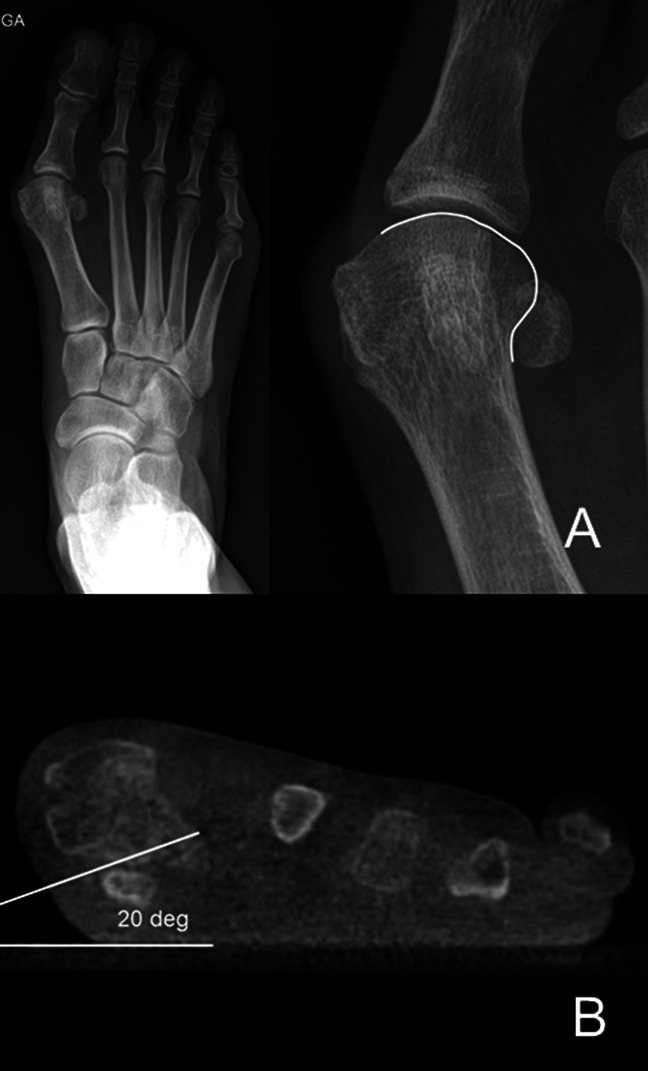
A, AP view of a hallux valgus case, where a magnification of the first metatarsal bone is on the right side of the image. The joint line is marked with a white line. If the joint line is followed onto the lateral aspect of the metatarsal head, it will represent the lateral metatarsal condyle, which gives the appearance of a rounded head. As in this case, the line is not “broken” but it is not possible to superimpose a perfect circle around the edge of the metatarsal; the predicted pronation is moderate (in the author's classification). B, Weight-bearing CT scan of the same patient presented in (A), where the measured pronation is 20°, between the horizontal weight bearing surface of the floor and the metatarso-sesamoid facets of the first metatarsal bone, confirming in this case a moderate pronation measurement (in the author's classification).
